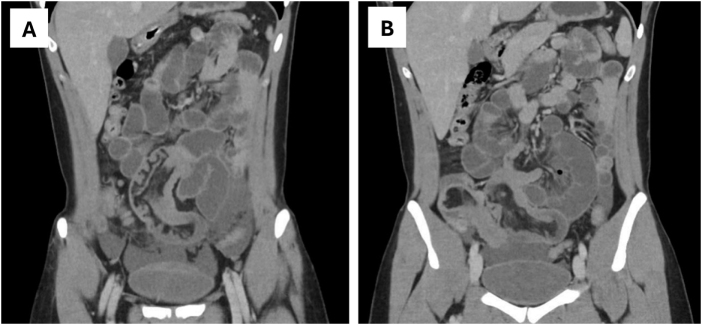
(A) Contrast-enhanced computed tomography showing dilated small bowel loops with a characteristic “bowel-within-bowel” configuration in the mid-abdomen, consistent with ileo-ileal intussusception. (B) Contrast-enhanced computed tomography demonstrating ileocolic intussusception with associated mild wall thickening of the distal ileum.
